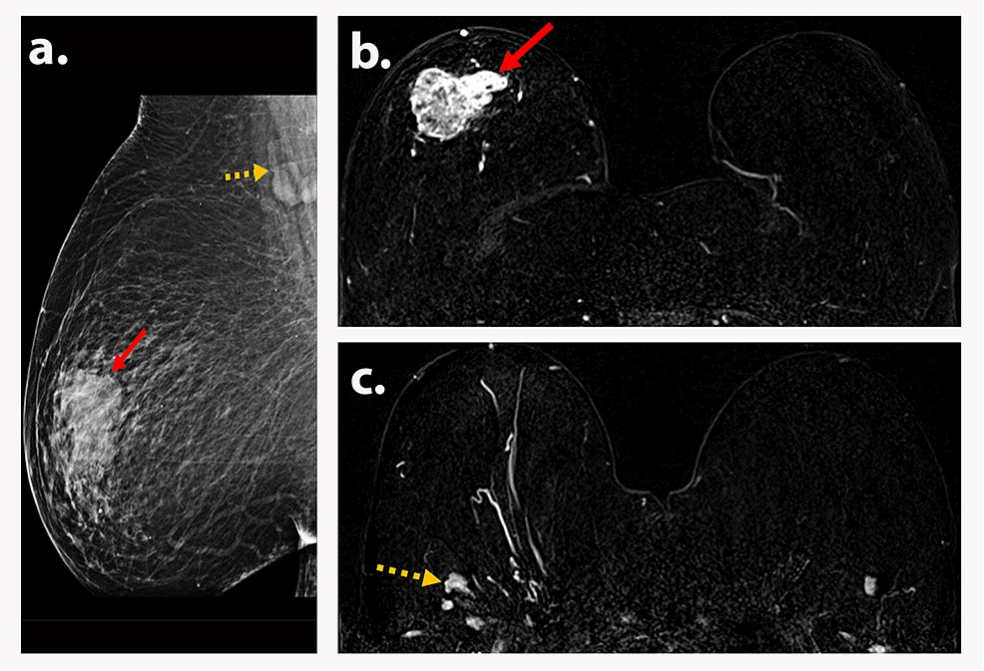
AJCC TNM stage IIIA (T3N1M0) A 40-year-old premenopausal woman presented with a palpable right breast mass. MLO of a screening mammography (a) shows a 6.6 cm irregular mass with indistinct and microlobulated margin (red arrow) in the superior right breast with axillary lymphadenopathy (yellow arrow). Axial T1-weighted DCE subtraction images (b and c) show a 6.6 cm irregular, heterogeneously enhancing mass (red arrow) in the upper outer quadrant of the right breast (red arrow) and associated level 1 axillary lymphadenopathy (dotted yellow arrow). Biopsy depicted invasive ductal carcinoma. Findings are consistent with AJCC TNM stage IIIA (T3N1M0). AJCC: American Joint Committee on Cancer; TNM: tumor, node, and metastasis; MLO: mediolateral oblique; DCE: dynamic contrast-enhanced.
